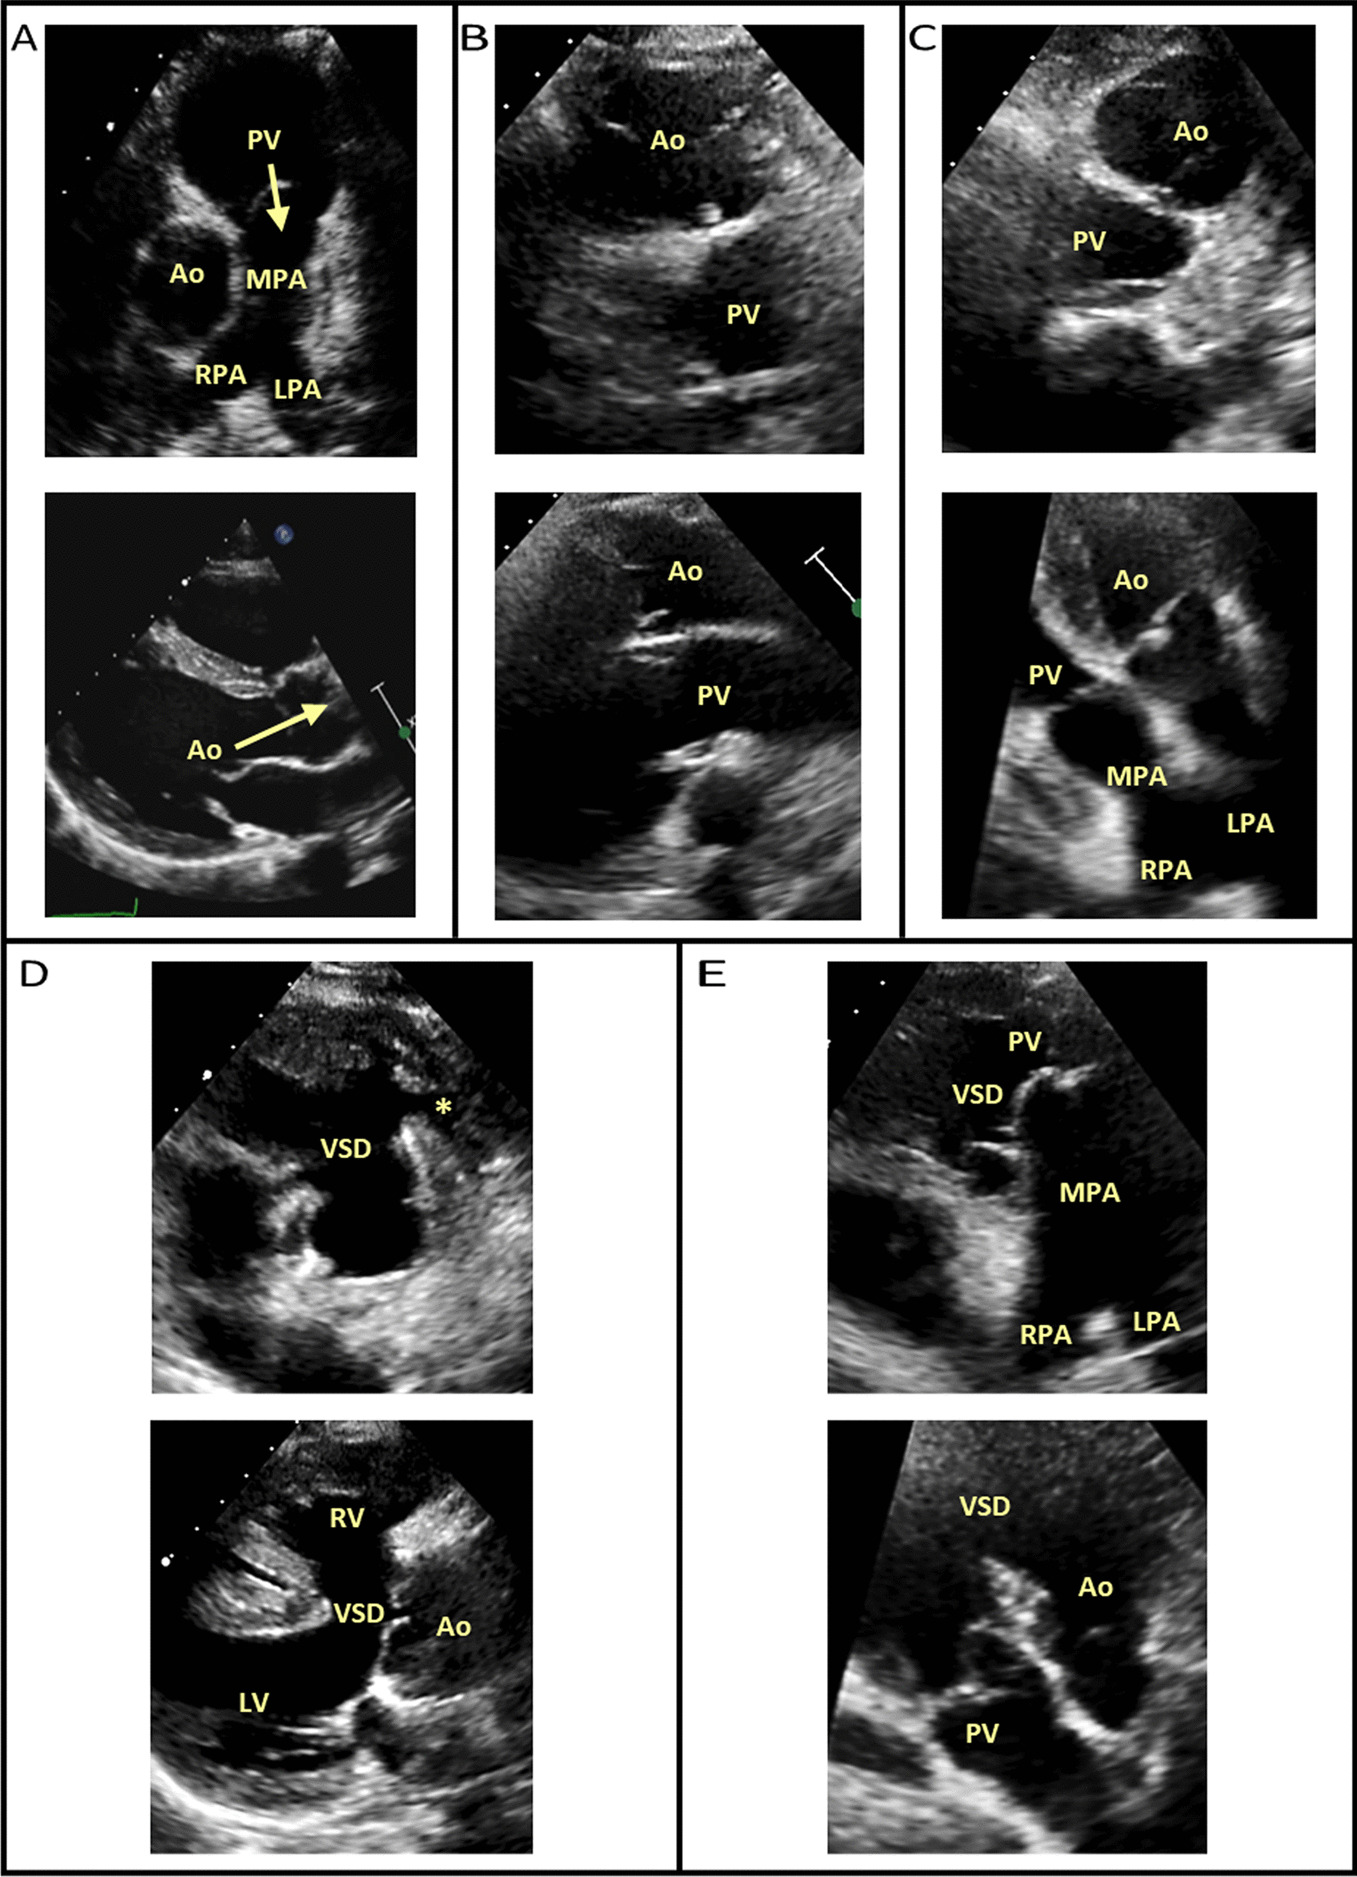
Potential ventriculoarterial (VA) connections examples. A Concordant VA connection (pulmonary outflow is anterior and leftward relative to the aorta (outflow roots cannot be viewed simultaneously, arrows identify respective outflow direction). B Discordant VA connection— transposition of the great arteries (aorta is anterior and rightward relative to the pulmonary valve in a parallel arrangement). C Discordant VA connection— congenitally corrected transposition of the great arteries (aorta is anterior and leftward relative to the pulmonary valve in a parallel arrangement). D Absent right VA valve/atretic pulmonary valve connection (*).From the parasternal window, differential diagnosis must be considered when a single overriding outlet is observed, for example pulmonary atresia, tetralogy of Fallot or truncus arteriosus. E Double outlet right ventricle. All images are examples from usual atrial arrangement (situs solitus) anatomies for convenience. Further variability can exist, which demonstrates the importance of sequential segmental analysis.
